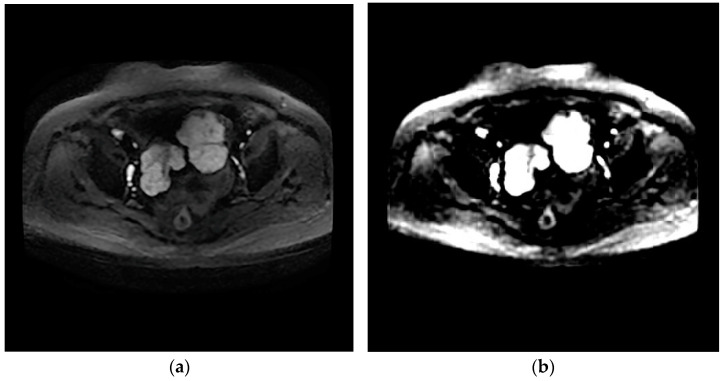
Axial DWI image (a); generated image (b).
